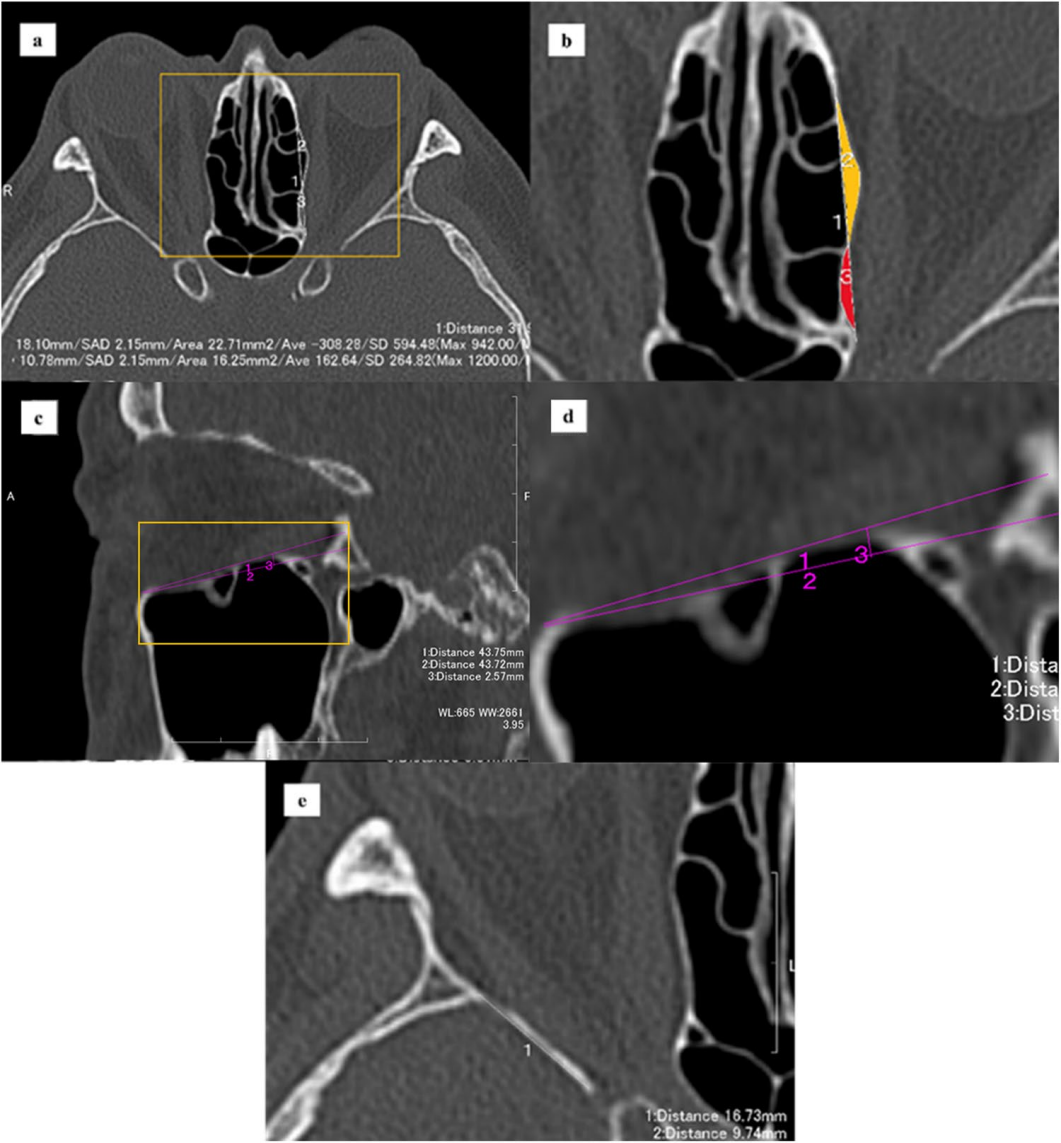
Evaluation of orbital wall configuration. a, b Axial computed tomography (CT) images showing the maximum cross-sectional area of the medial rectus muscle and bulged (yellow area in b) and dented (red area in b) areas in the medial orbital wall. b is a magnification of the rectangular portion in (a). c, d A line was drawn on the quasi-sagittal CT image through the optic nerve to connect the inferior orbital rim and the orbital process of the palatal bone. The length of the perpendicular distance between that line and the tip of the superior bulge of the orbital floor (length of the superior bulge) was then measured. d is a magnification of the rectangular portion in (c). e The distance between the posteromedial vertex of the trigone and the superior orbital fissure was measured
